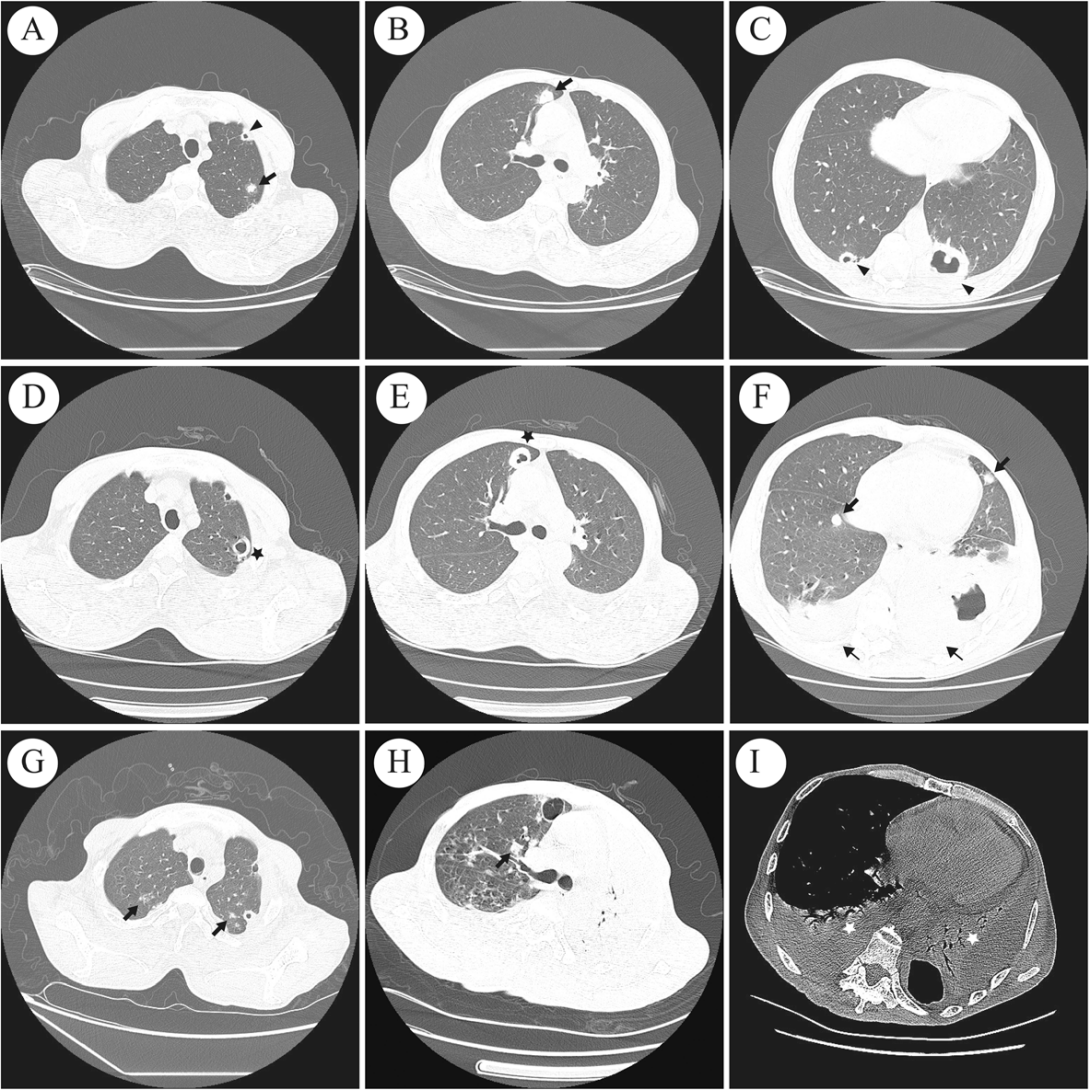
Chest CT findings during hospitalization. a-c: A CT scan of the chest (on admission) showing multiple nodules (thick arrows) and thick-walled cavities (black triangle) in lung fields as well as enlarged mediastinal lymph nodes. d-f: Subsequent chest CT (23rd day) showing new emerging round opacities (thick arrows), expending lung abscess and cavities (black stars), bilateral pleural effusion (thin arrows). g-i: Subsequent chest CT (49th day) showing increased pleural effusion, atelectasis and consolidations on both sides with air bronchograms (white star)
